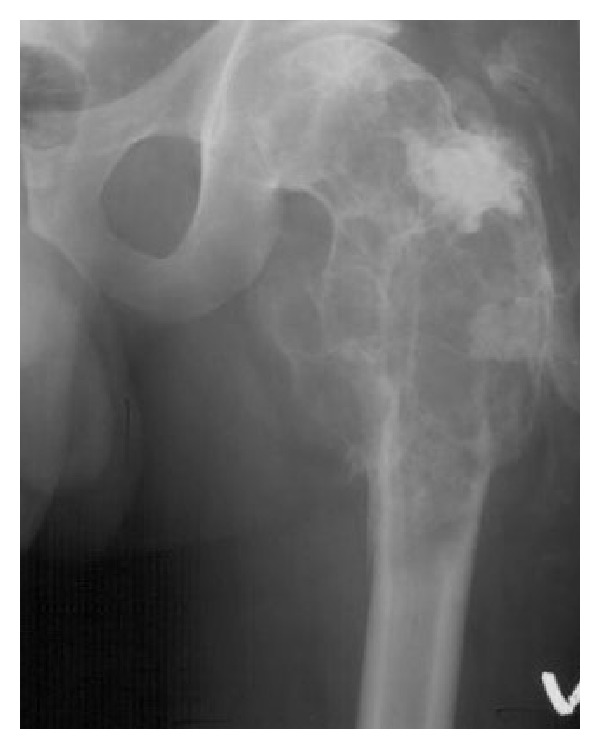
Radiograph from the original tumour.
